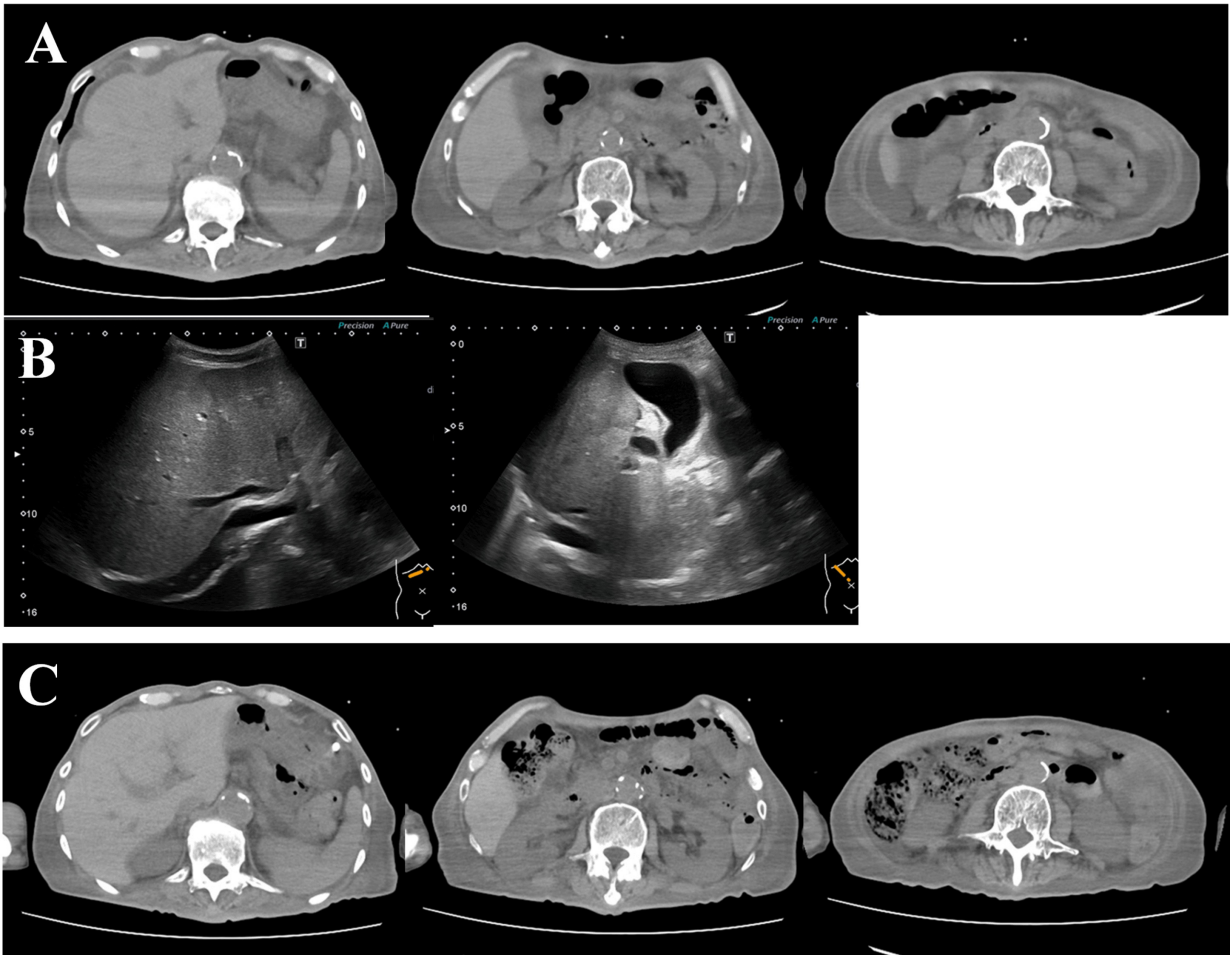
Before and after administration of prednisolone (A) On day 115, computed tomography revealed fluid accumulation in the bowel, ascites, and subcutaneous edema over the trunk due to hypoproteinemia. (B) Abdominal ultrasonography showed no inflammation in the liver or gall bladder. (C) Abdominal computed tomography on day 142 showed the accumulation of solid stool in the ascending and transverse colon and a decrease in the ascites.
